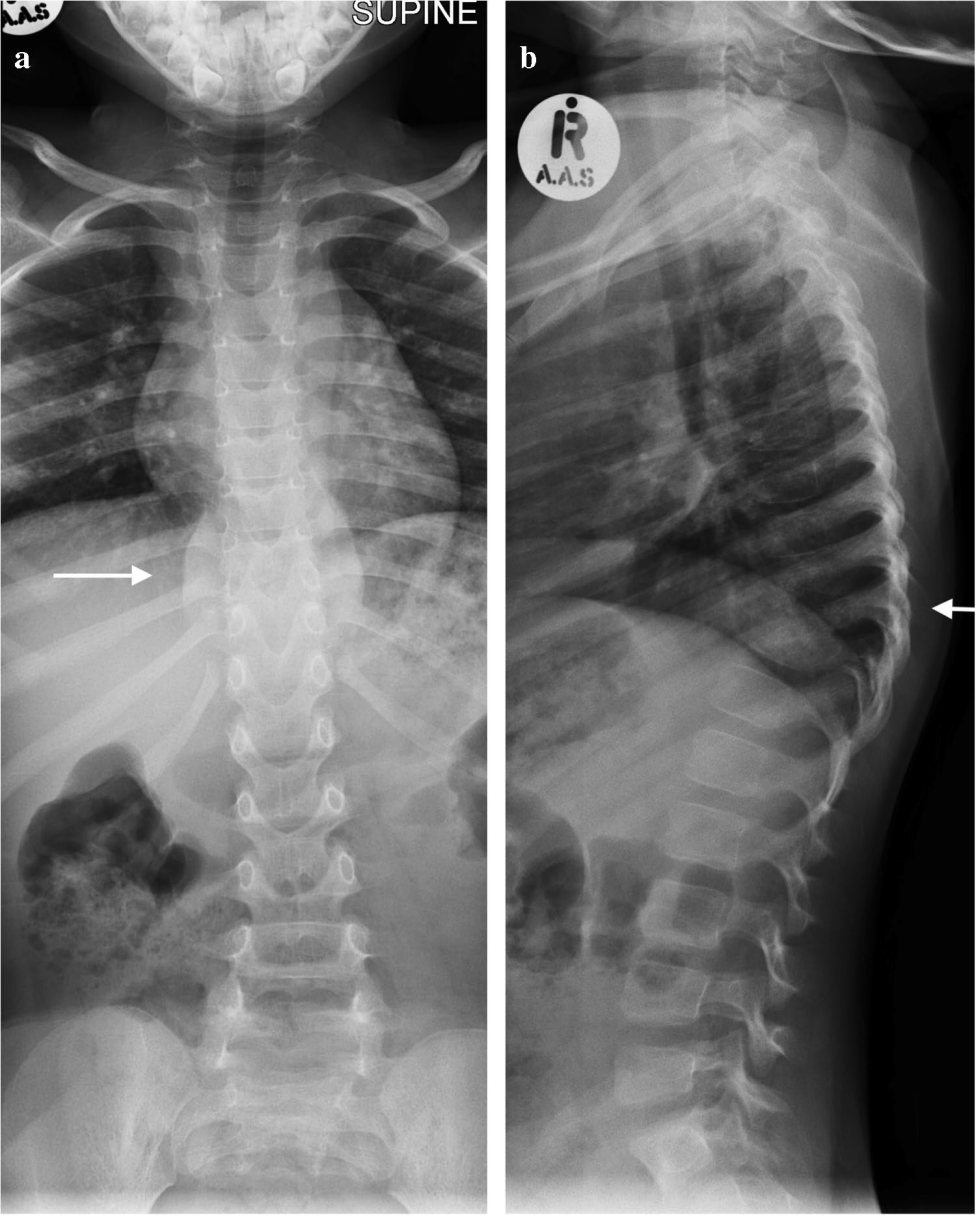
Spine radiographs in a 3-year-old boy who presented with a lump on his back and in whom tuberculosis was confirmed. a The anteroposterior radiograph shows a  paravertebral soft tissue mass extending from T8 to T11 (*arrow*). b The lateral radiograph shows a thoracic kyphosis due to collapse of the T10 vertebral body (*arrow*)
